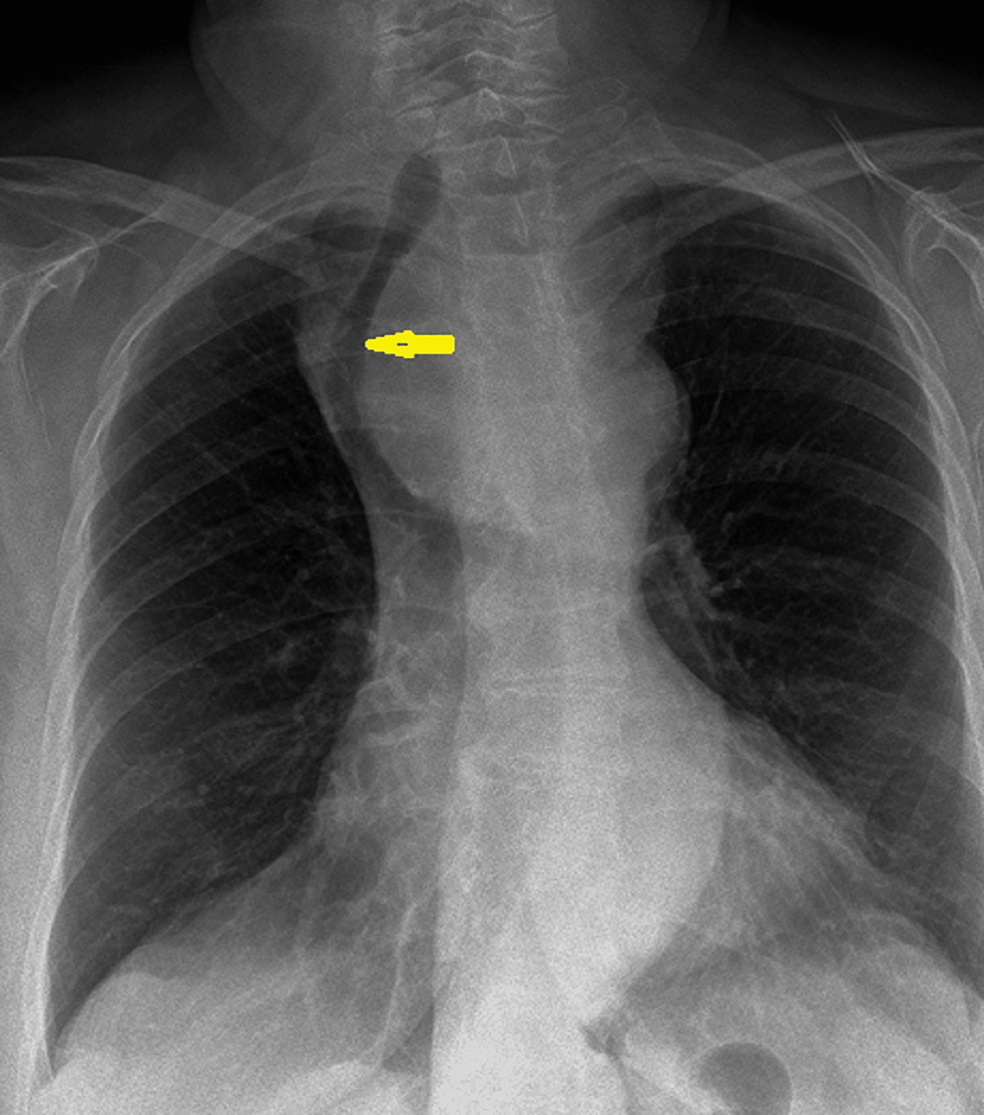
Radiography x-ray showing retrosternal thyroid mass causing tracheal deviation (arrow).
